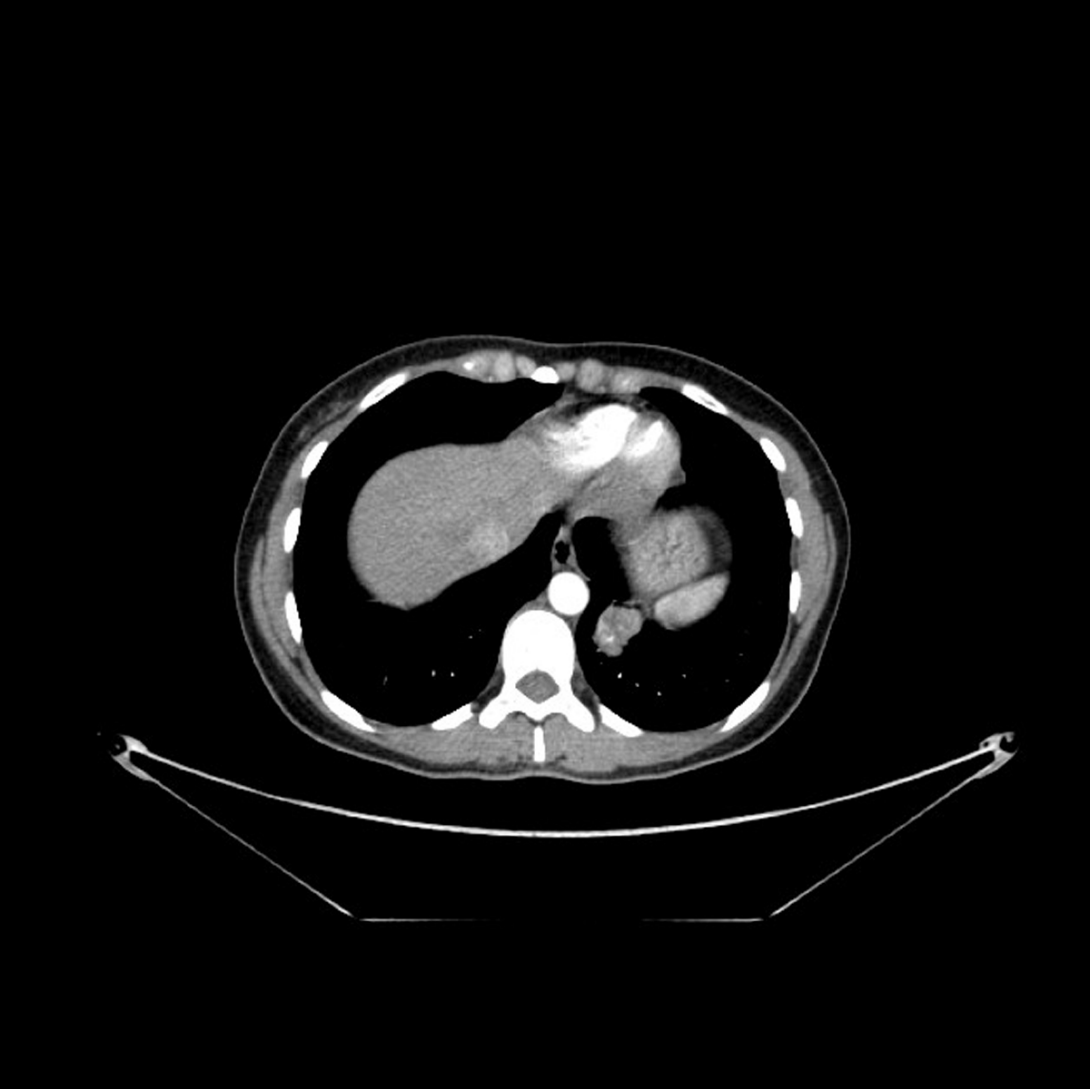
Chest computed tomography (CT) scan demonstrated an 4 cm pulmonary mass in the left lower lobe, without enlargement of intrapulmonary or mediastinal lymph nodes.
